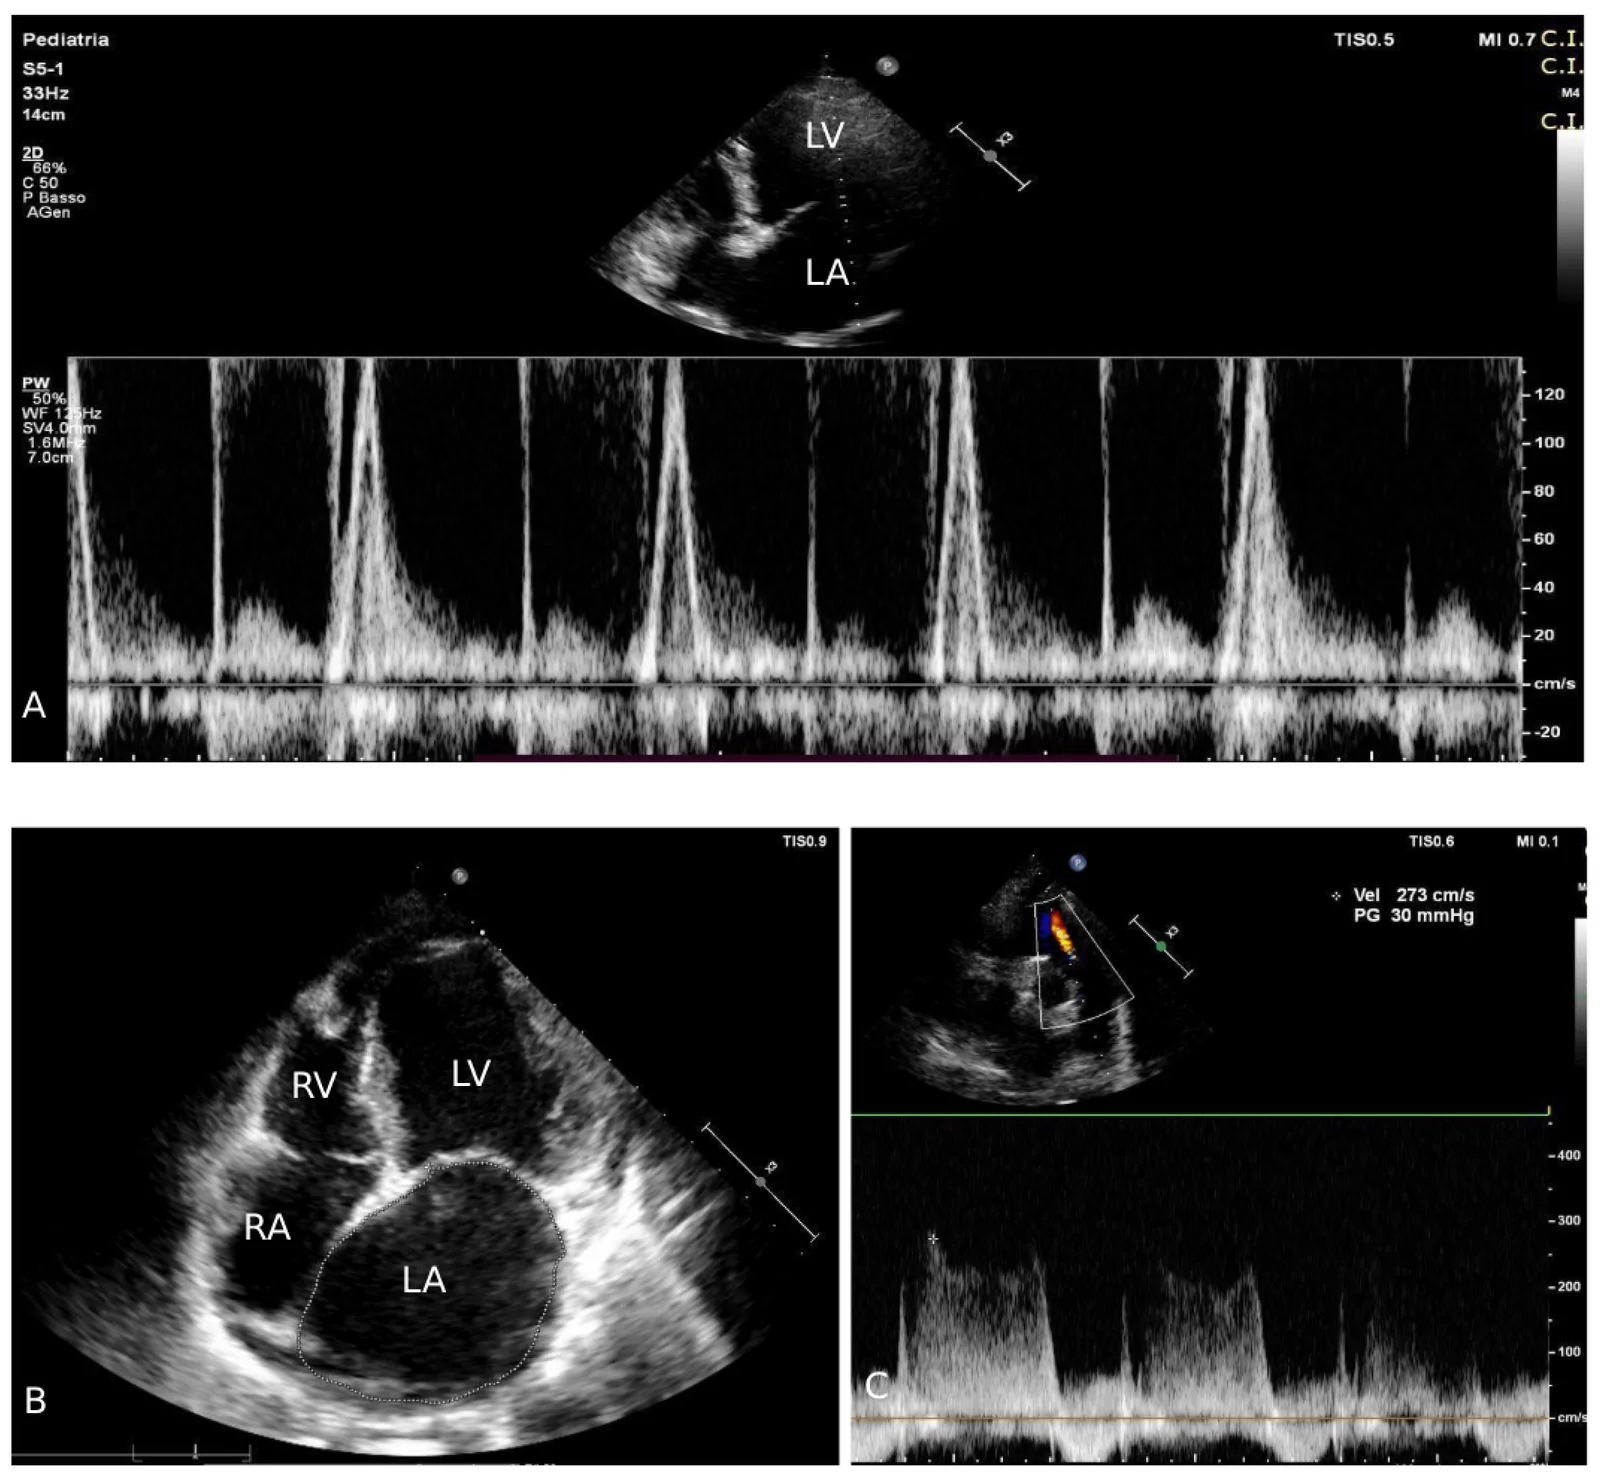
Transthoracic echocardiography (TTE) performed during the acute postpartum decompensation (Case 2). (A) Transmitral pulsed-wave Doppler demonstrating a restrictive filling pattern, with an E/A ratio of 2.4. (B) Apical four-chamber view highlighting significant left atrial enlargement, reflecting the retrograde transmission of elevated filling pressures from the rigid, truncated left ventricle. (C) Continuous-wave Doppler of the pulmonary regurgitation envelope used to estimate the mean pulmonary artery pressure (mPAP), confirming mild post-capillary pulmonary hypertension.
